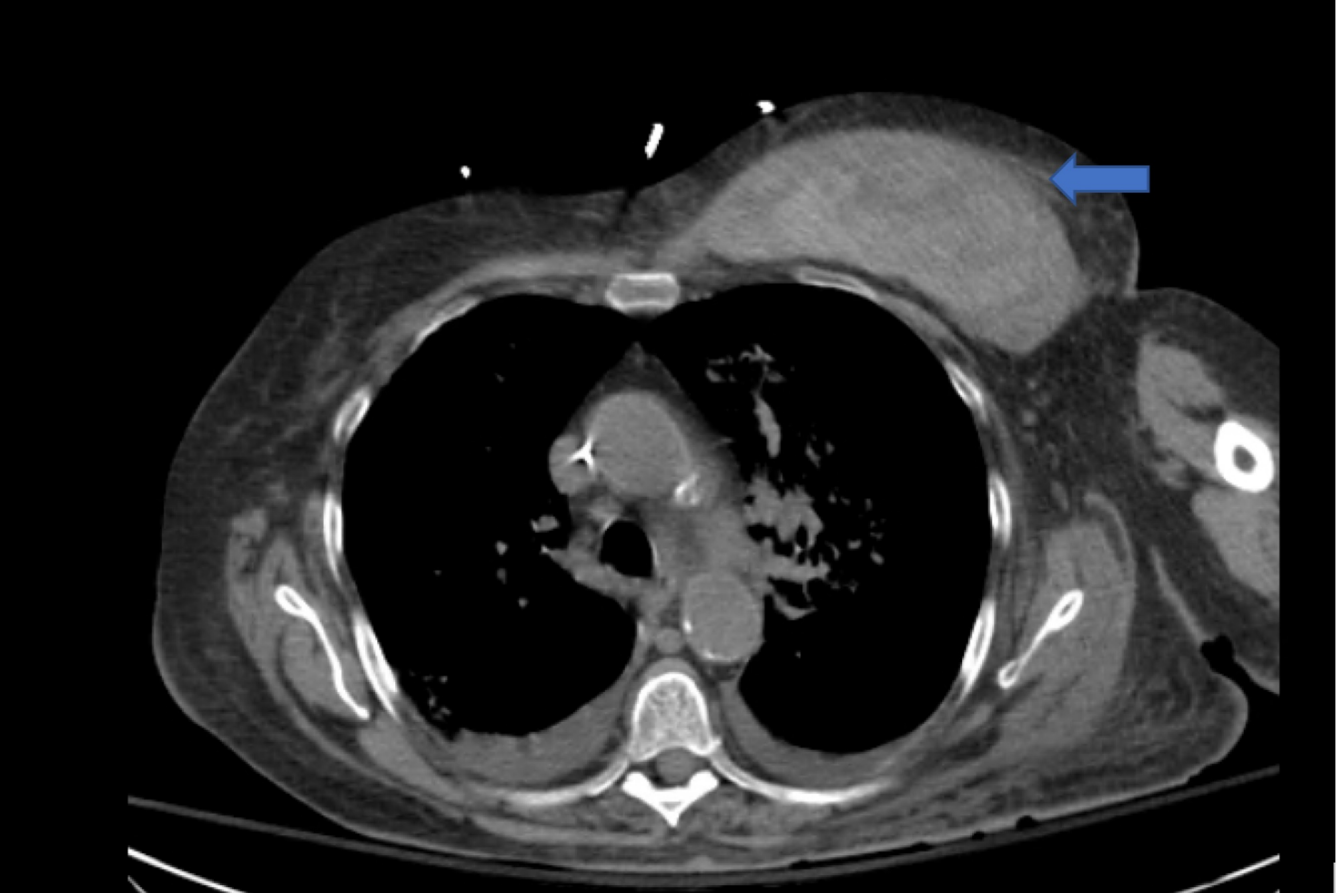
CT angiogram showing left pectoral hematoma
